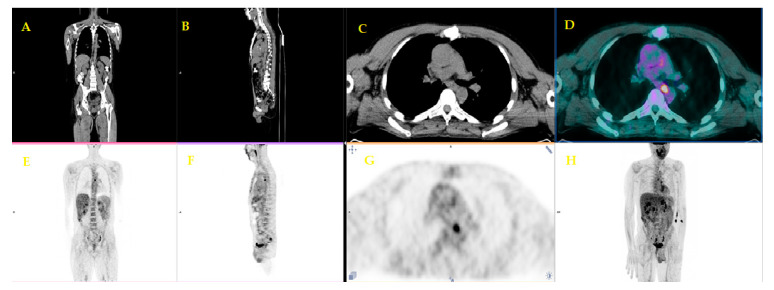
Lymph-nodal uptake suggestive of metastasis in the same patient (Images from Nuclear Medicine Unit, Padova University Hospital). Legend: A = Coronal image of low-dose CT; B = Sagittal image of low-dose CT; C = Axial image of low-dose CT; D = PET/CT fused image; E = Coronal image of PET; F = Sagittal image of PET; G = Axial image of PET; H = Maximum intensity Projection (MIP).
